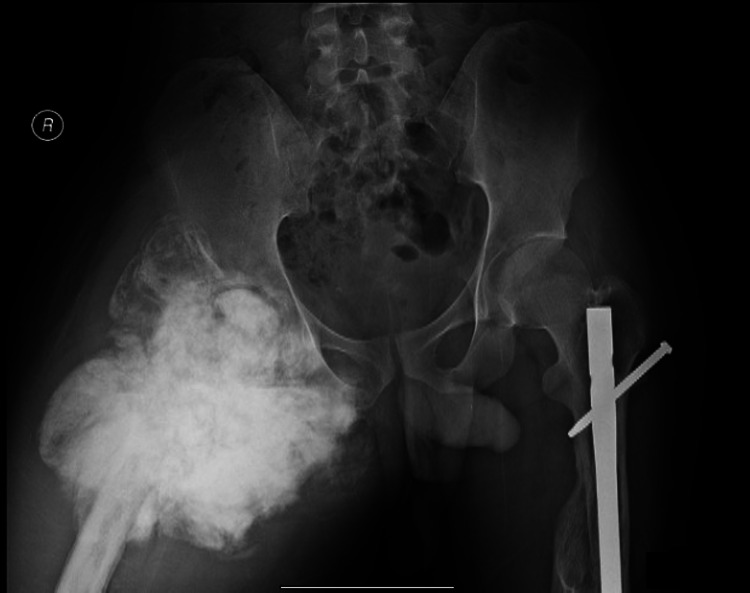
The plain radiograph one year before the patient succumbed to death There was an extensive involvement of the proximal right femur with no evidence of a similar lesion within the visualized contralateral femur. The left femoral nail remained in situ. R: right.
